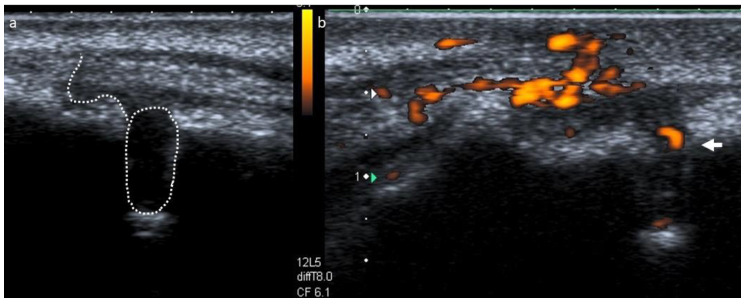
(a) B-mode ultrasound examination showing a sinus tract disrupting the vestibular bone, presenting as a dishomogeneous, hypoechoic pathway lined by echogenic and reinforced contours. (b) Power Doppler applied to the same area, showing an echogenic, solid lesion with poorly defined hyperechoic bone boundaries and internal blood vessels.
